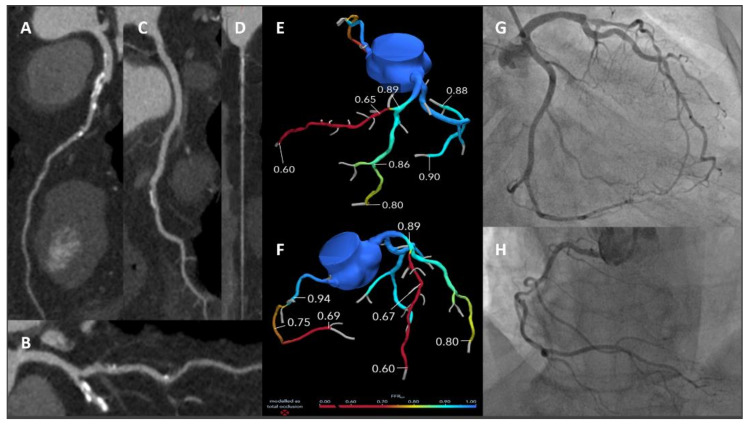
A 59-year-old male smoker with hypertension and dyslipidaemia presented with several episodes of atypical chest pain. A cCTA was prescribed. Panels (A–D) showed critical stenosis at the mid LAD (A), moderate stenosis at the main diagonal branch (B), mild calcific stenosis at the main obtuse marginal branch (C) and significant stenosis at the mid RCA (D). Panels (E,F): FFR_CT_ analysis (HeartFlow Inc.) showing haemodynamically significant LAD and RCA stenoses. Panels (G,H): ICA showing severe stenosis at the mid LAD, mild stenosis of the main diagonal branch and main obtuse marginal branch (G) and significant stenosis of the mid RCA (H). *cCTA: cardiac computed tomography angiography; LAD: left anterior descending; RCA: right coronary artery; FFR_CT_: fractional flow reserve from computed tomography; ICA: invasive coronary angiography.*
